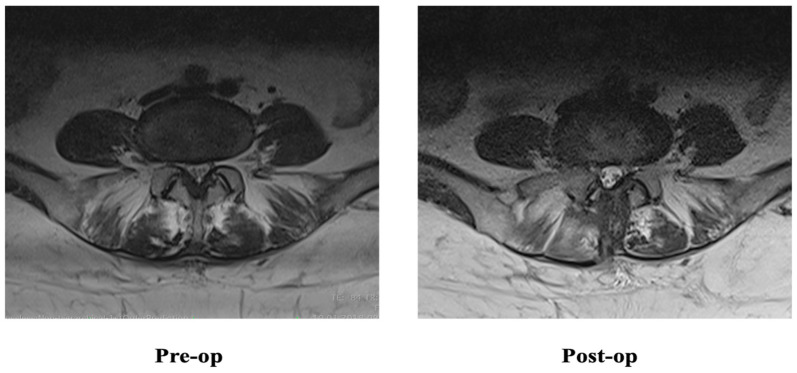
Changes in multifidus muscles on magnetic resonance image after microlumbar discectomy.
